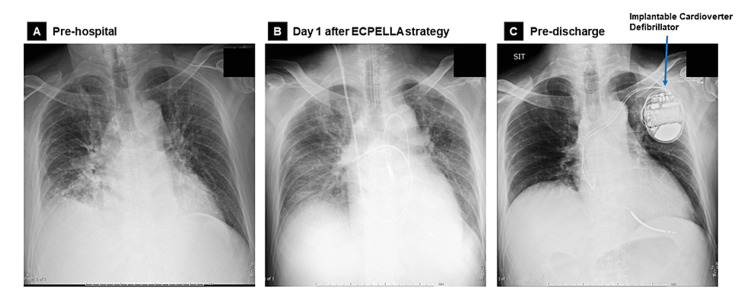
Progress of chest X-ray Chest X-ray at discharge (C) showing improvement in pulmonary congestion that had been observed in the previous hospital (A) and day 1 after insertion of ECPELLA (combined configuration of VA-ECMO and Impella) (B).
